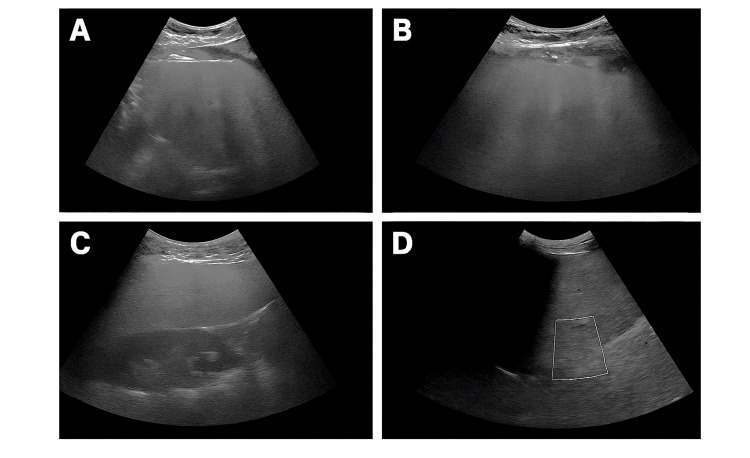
Ultrasound showing nodular liver surface with features suggestive of cirrhosis Ultrasound imaging of the liver demonstrating nodular surface architecture and heterogeneous echotexture, features suggestive of cirrhosis. Multiple grayscale images show surface contour irregularities, with panel D highlighting a region of interest.
